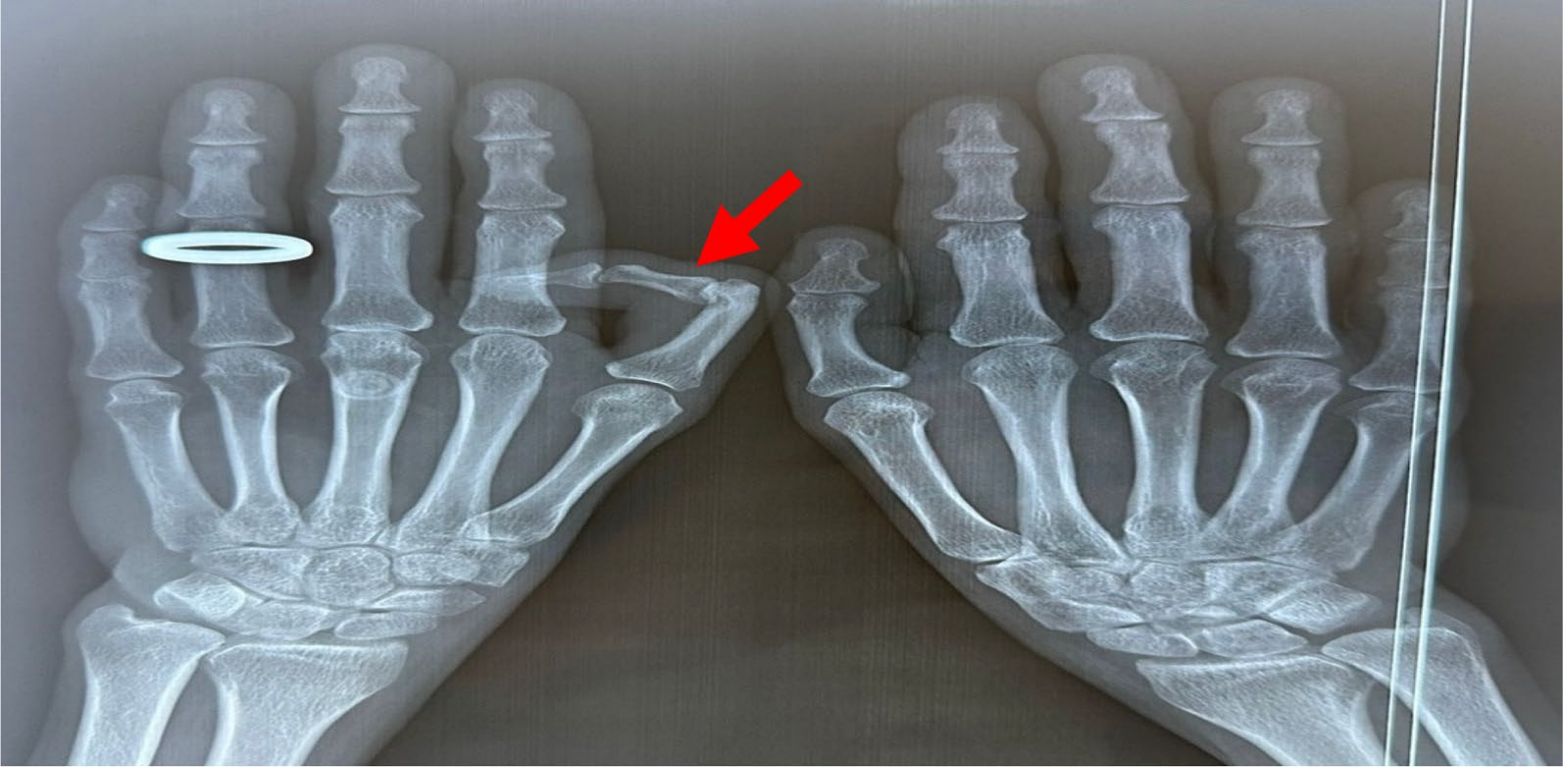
Hand X-rays confirming a triphalangeal left thumb (rogue arrow)
